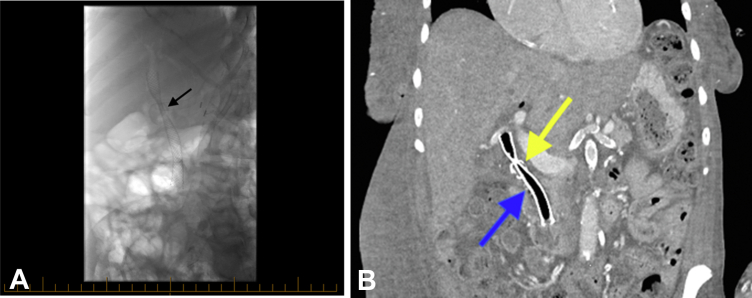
A, Fluoroscopy image following insertion of a self-expandable metal stent (SEMS) through the common bile duct (CBD) stone (*black arrow*). B, Abdominal CT scan (*coronal*) showing SEMS (*blue arrow*) passing through the CBD stone (*yellow arrow*).
